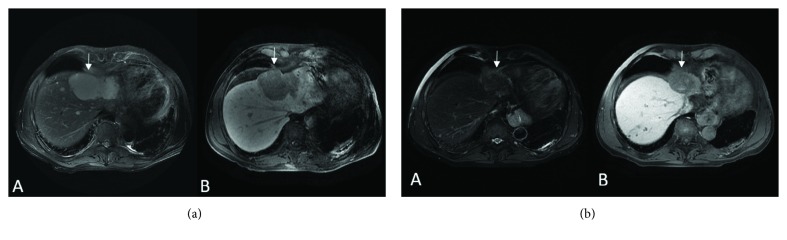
(a) Pretreatment scan (8/22/2016) (MRI). Axial T2 WI (A) and postcontrast (Eovist) Axial T1 WI (B) showing an 11 cm mass in the left liver lobe (arrows) and multiple smaller lesions in both liver lobes. (b) Ongoing treatment (5/2/2018) (MRI). Axial T2 WI (A) and postcontrast (Eovist) Axial T1 WI (B) show the decrease in size of the largest mass in the left liver lobe (arrows) now measuring 4.8 cm with no enhancement. There is a decrease in size and number of the multiple smaller lesions in both liver lobes.
